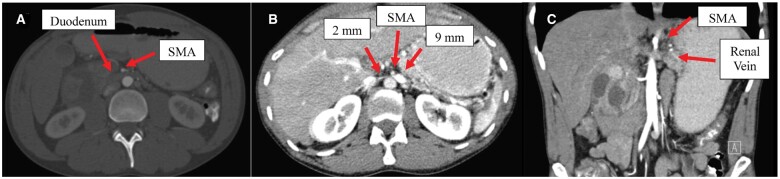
Image of superior mesenteric artery syndrome and two images of renal vein entrapment syndrome. (A) Contrast is not easily passing through into the second and third portion of duodenum. The third portion the duodenum does appear narrowed as it extends under the superior mesenteric artery (SMA). (B) This axial CT image shows atypical venous drainage of the left kidney with appearance of a nutcracker syndrome. In this view, the renal vein to the left of the SMA appears dilated measuring up to 9 mm. The renal vein more medial to the SMA is thin and strand-like measuring 2 mm. This could be a hemodynamically significant stenosis. (C) This CT coronal slice is another view of nutcracker syndrome evident in the patient.
